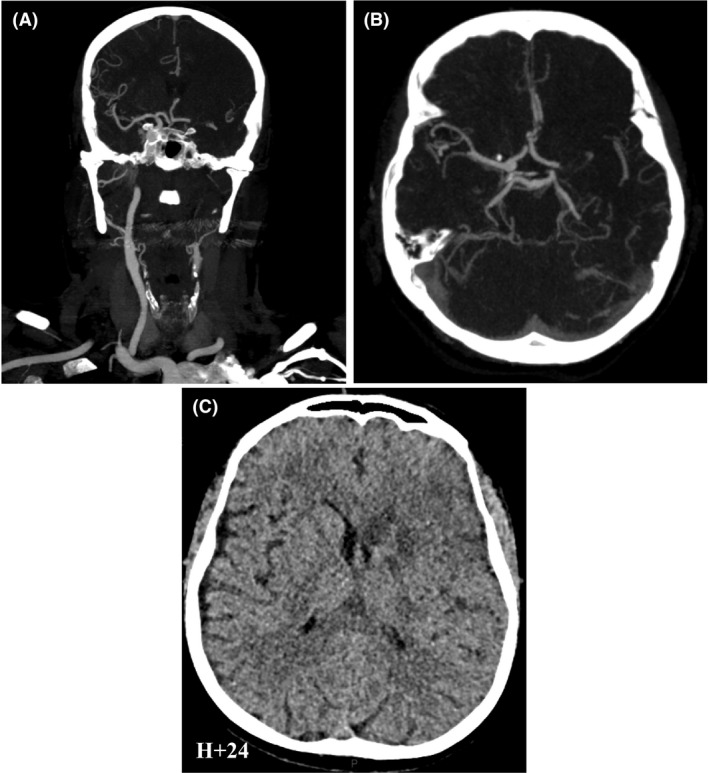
Cerebral imaging. (A) Head and neck CT angiography in acute setting showed an absence of opacification of the cervical segment of the left internal carotid artery. (B) Head CT angiography in acute setting revealed a hyperacute middle cerebral artery occlusion. (C) Follow‐up noncontrast CT on day 1 showed a left lenticulostriate infarct without hemorrhagic transformation.
